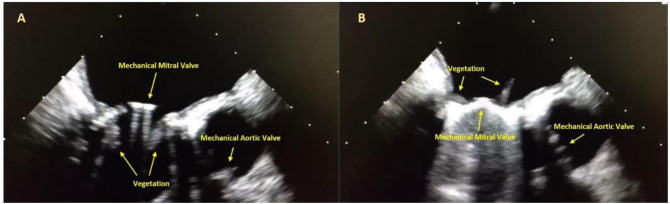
A, B. Transesophageal echocardiography examination in 120° showed mechanical prosthetic bileaflet mitral valve with multiple large size vegetation (arrow) on both sides of prosthetic valves.
